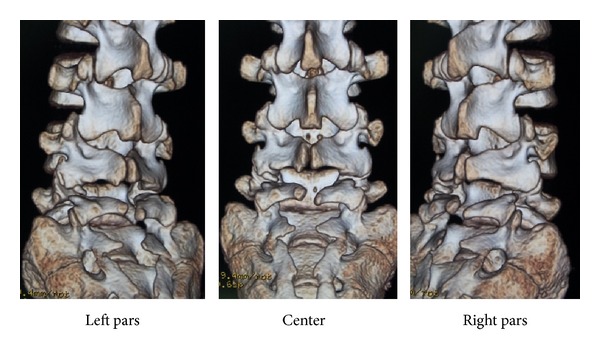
Three-dimensional computed tomography (3D-CT) of Case 1. Note the concomitant existence of L5 bilateral spondylolysis and SBO.
